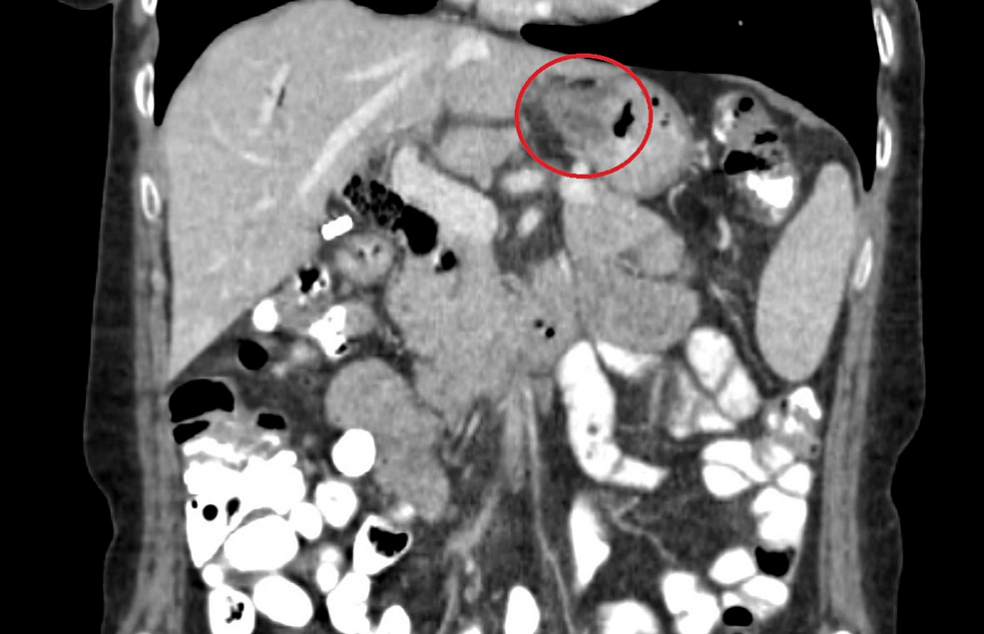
CT scan of the gastric mass
